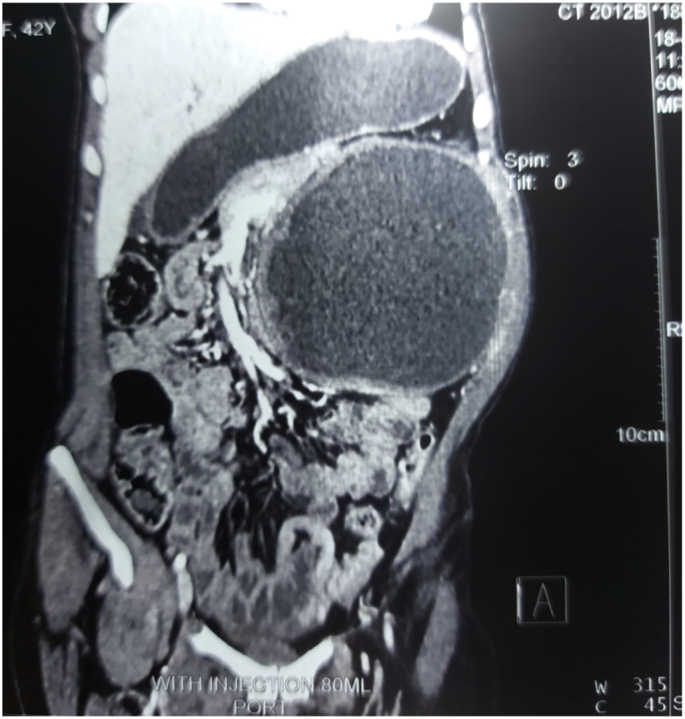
Coronal section of abdominal CT scan showing low attenuating well defined in the left upper quadrant of abdomen with mild hydronephrosis left pelvi-calyceal system.
